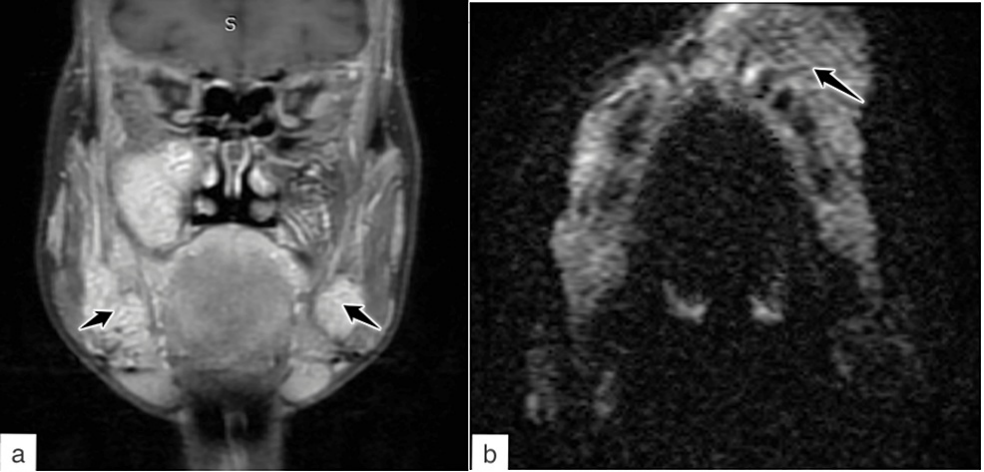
MRI face and neck – (a) coronal T1W post-contrast and (b) DWI. The lesions show post-contrast enhancement and restricted diffusion.
